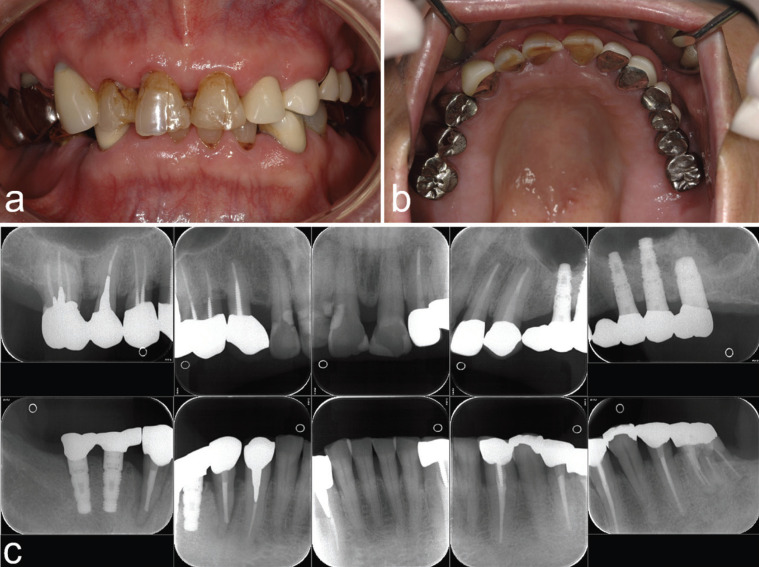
Intraoral views and dental X-ray photos in 2022 at 20 years after sinus augmentation. a: deep bite. b: view of maxilla. c: whole view of dental X-ray photos.
